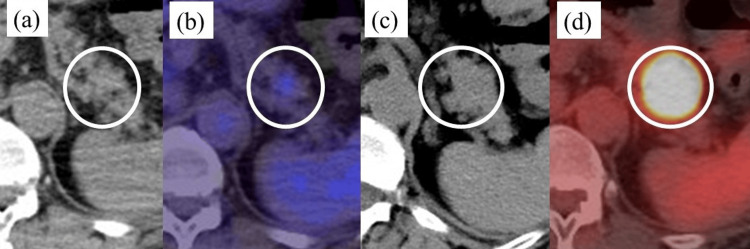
Abdominal plain computed tomography (CT) and 18F-fluorodeoxyglucose (FDG) positron emission tomography (PET)/CT (a-b) At the onset of tuberculous lymphadenitis. (c-d) Three months after the onset of lymphadenitis (during the first visit to our department). (a and c) Abdominal CT. (b and d) 18F-fluorodeoxyglucose (FDG) positron emission tomography (PET)/CT. At the onset of tuberculous lymphadenitis, 18F-FDG PET/CT confirmed a mild uptake area in the pancreatic tail (SUVmax = 2.8). Three months after the onset of lymphadenitis, this area enlarged in plain abdominal CT, and PET/CT confirmed increased uptake in this area (early SUVmax = 37.37, delayed SUVmax = 50.81). SUVmax: maximum standardized uptake value.
